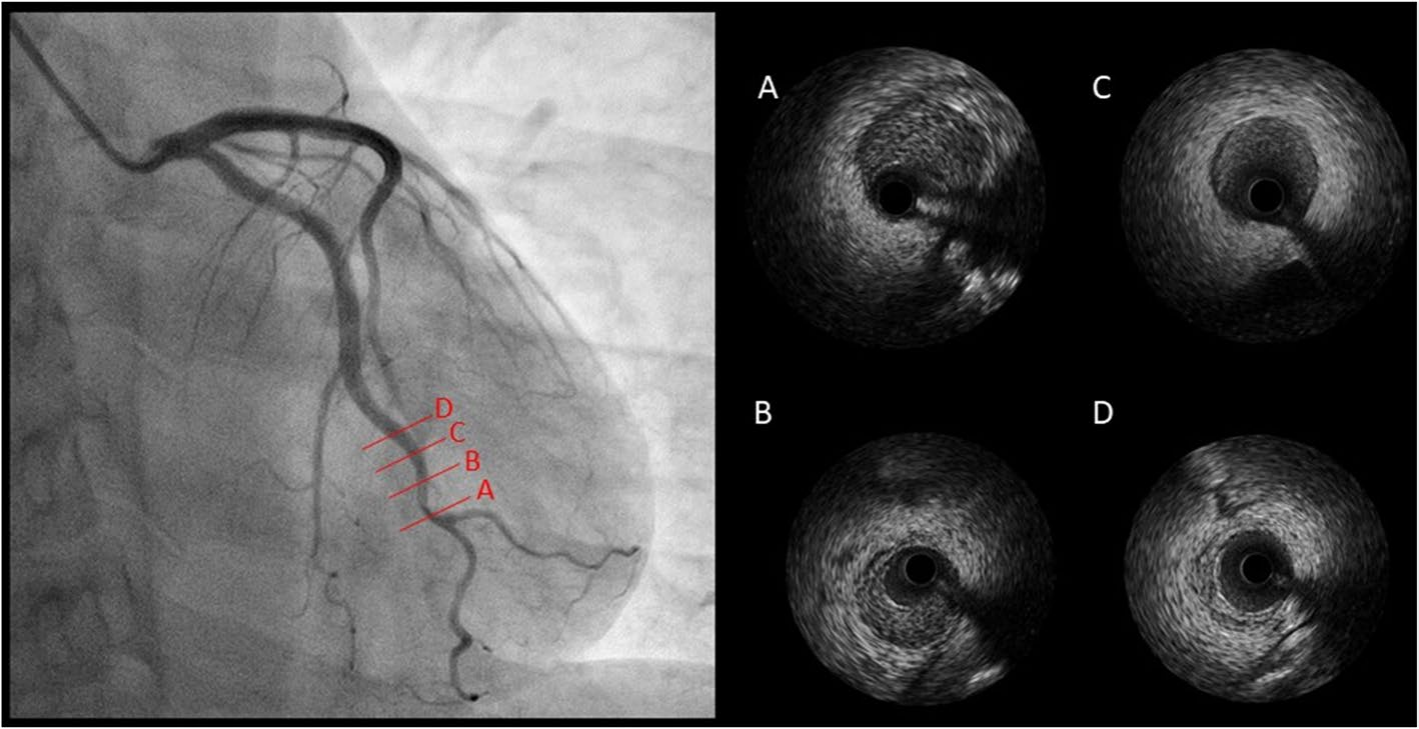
SCAD lesion imaged at four levels in the LCX. The red lines A, B, C, D in the left panel correspond with the images in the right panel. We diagnosed an intramural haematoma with observed heterogeneity on IVUS. SCAD, spontaneous coronary artery dissection; LCX, left circumflex artery; IVUS, intravascular ultrasonography
